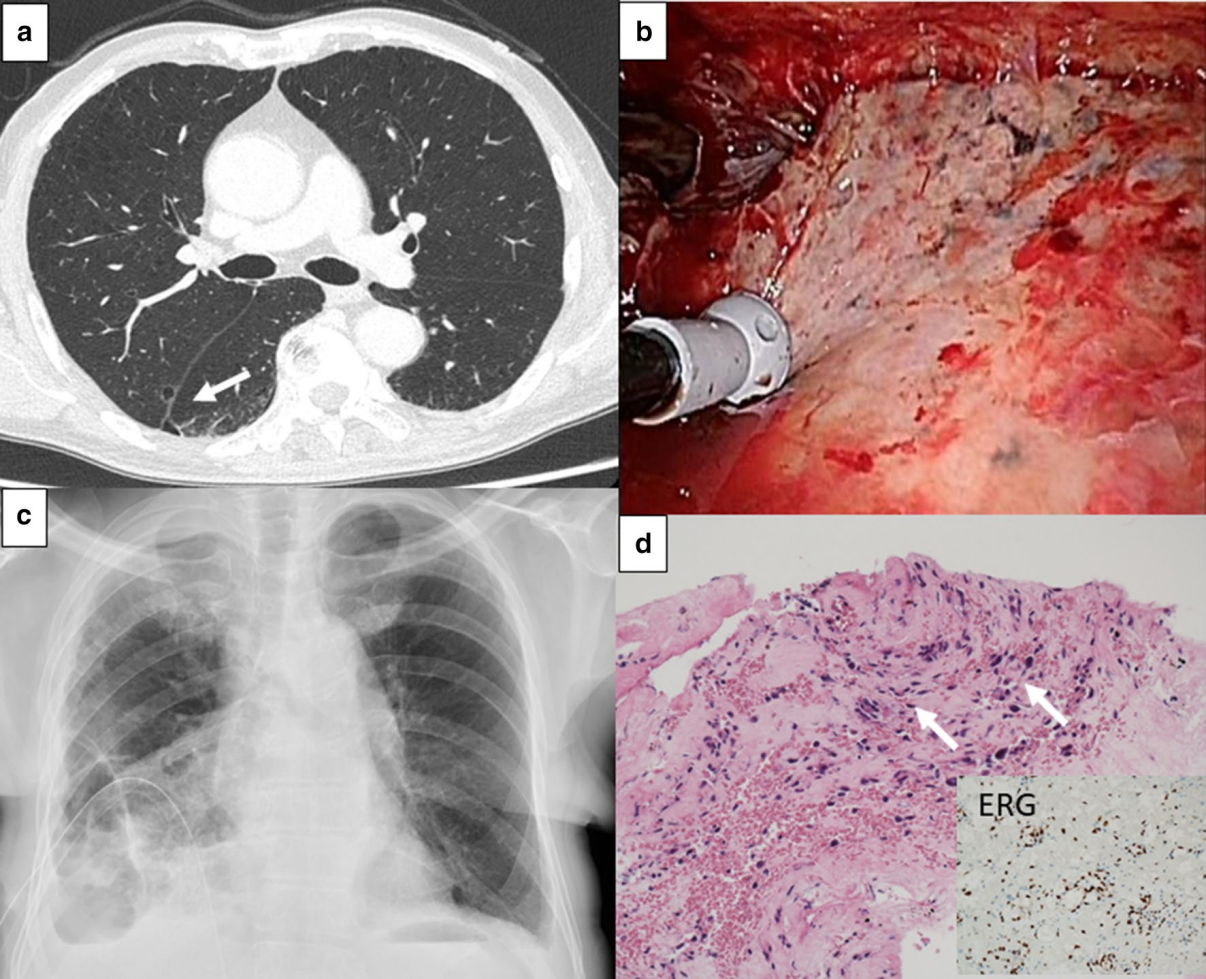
a CT, performed 2 months before diagnosed with pneumothorax, showed bullae lesions at the edge of the right upper lobe and right lower lobe (arrow). b Intraoperative findings demonstrated fibrinopurulent empyema. c Chest X-ray showed expanded right lung; however, dark-red pleural effusion of more than 500 ml per day continued. d Pathological findings showed atypical spindle-like cells infiltrating the parietal pleura (arrows). Erythroblast transformation-specific-related gene (ERG) was positive in tumor cells, suggesting the differentiation of endothelial cells
